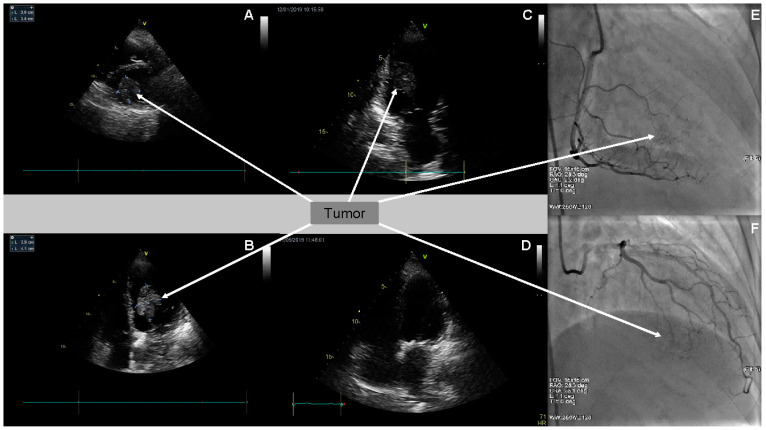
Coronary angiography (CA) and transthoracic echocardiography (TTE). (A) CA (RAO 28.3°, CAU 2.2° presentation)—visible poor tumor vascularity at the late arterial phase of the right coronary artery selective angiography (arrow); (B) CA (RAO 26.3°, CRA 23.1° presentation)—visible poor tumor vascularity at the late arterial phase of the left coronary artery (circumflex branch) selective angiography (arrow). (C) Two-dimensional TTE (parasternal, short axis presentation)—tumor in the left ventricle cavity (arrow); (D) 2D TTE (apical 4-Ch presentation)—tumor in the left ventricle cavity (arrow); (E) 2D TTE (2-Ch presentation)—tumor in the left ventricle cavity (arrow); and (F) 2D TTE (4-Ch presentation)—tumor resolution after chemotherapy.
